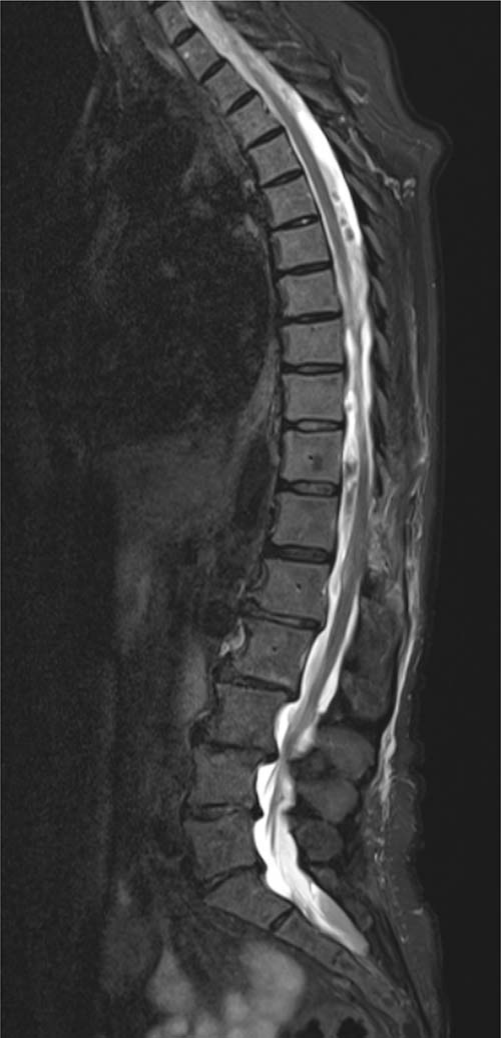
Follow-up MRI; it demonstrates a partial resolution of the hematoma with a confined area of myelopathy at the T12 level.
